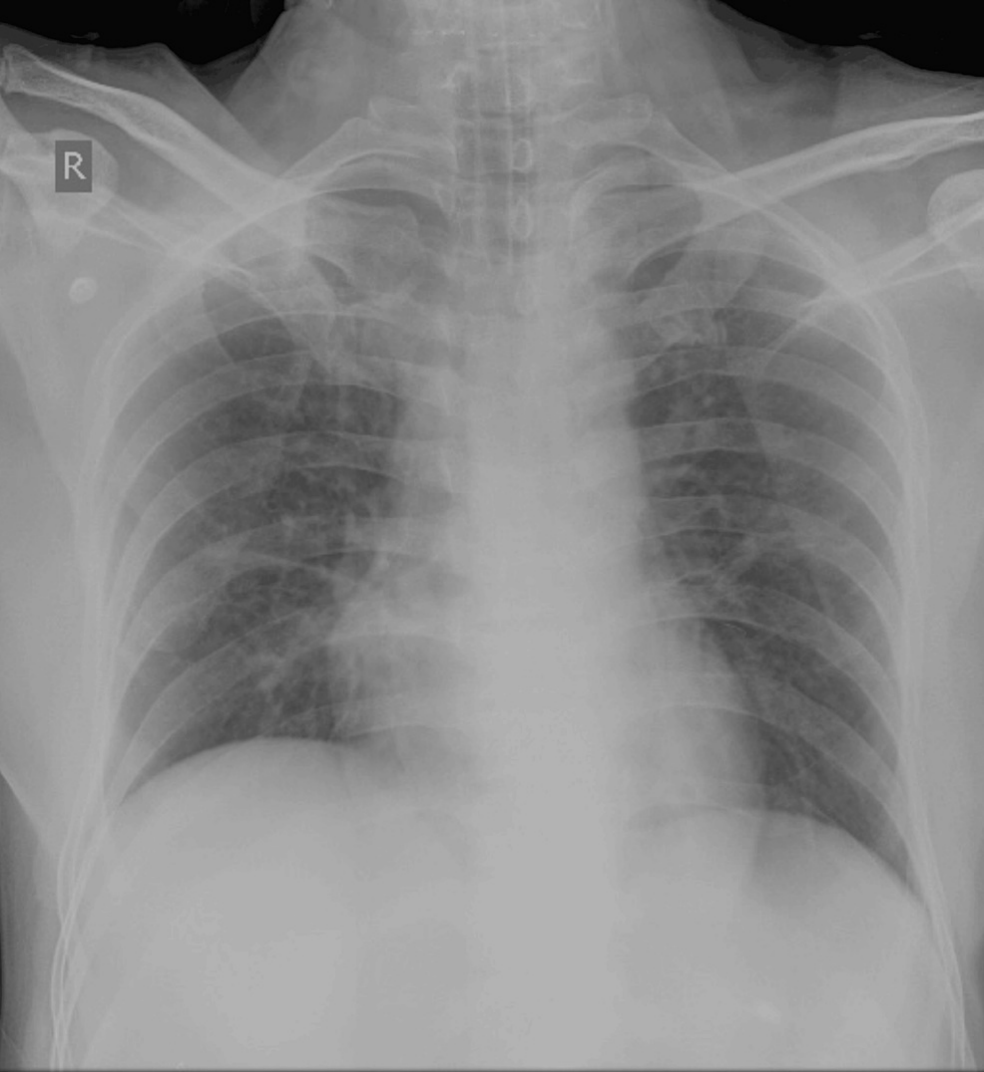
Chest radiography showing no significant abnormality and an endotracheal tube in situ.
